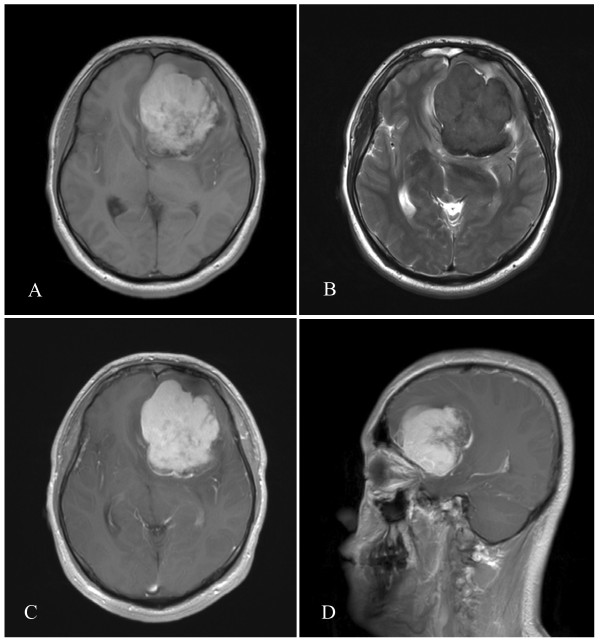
Preoperative MR images showing the left frontal tumor with lobulation, ventricle compression and midline shift. A: Axial view. The lesion appears hyperintense on T1-weighted images. B: Axial view. Hypointense signals are noted on T2-weighted image of the brain. C: Axial post contrast T1-weighted view. Contrast enhancement of the tumor. D: Sagittal view. Adhesion of the tumor to the meninges of the anterior cranial fossa (arrow) MR, magnetic resonance.
